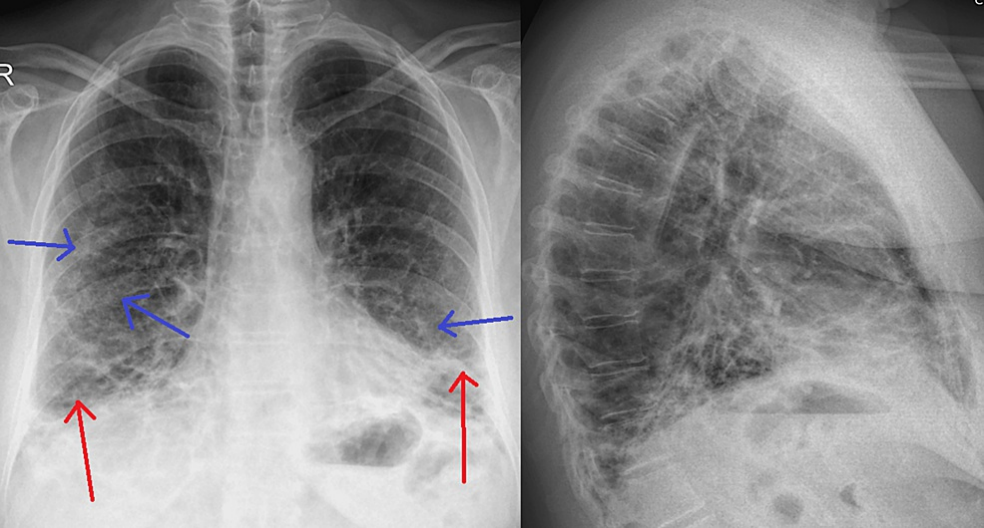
Admission chest X-ray Asymmetrical basal predominant reticular pattern (red arrows) with few areas of alveolar infiltrates (blue arrows) bilaterally.
